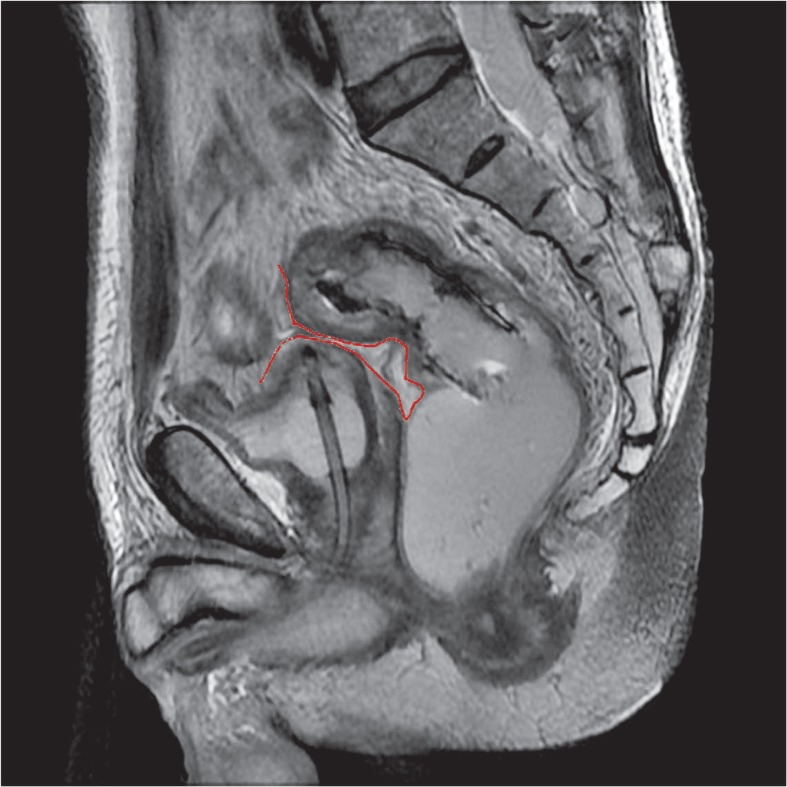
Sagittal view of the peritoneal reflection (red line) by rectal MRI.
